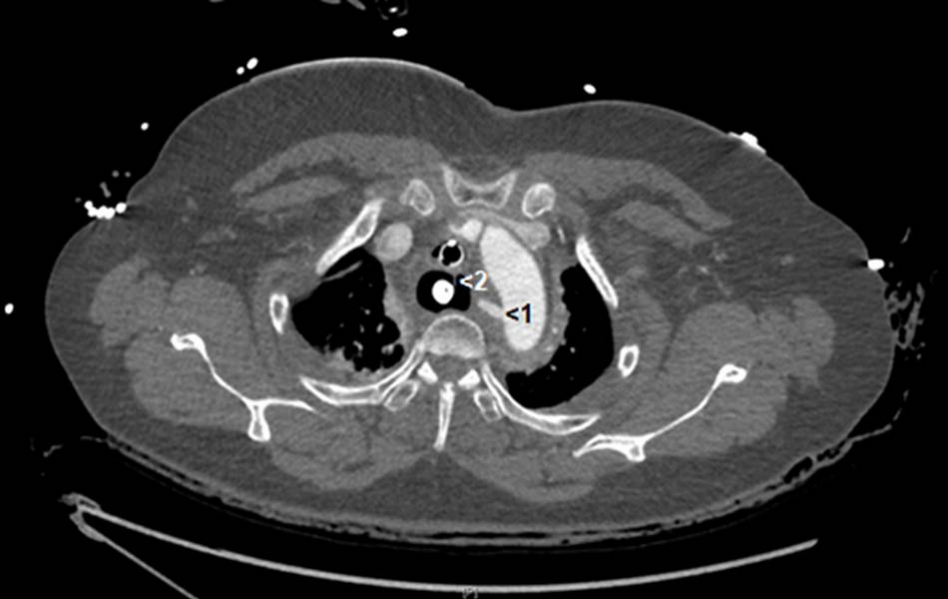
Axial CT angiograph. This image demonstrates the unusual arterial fistula (red number 1) connecting the arch of the aorta to the oesophagus. A Blakemore tube with inflated oesophageal balloon (red number 2), used to tamponade the arterial haemorrhage, can be seen within the oesophagus.
